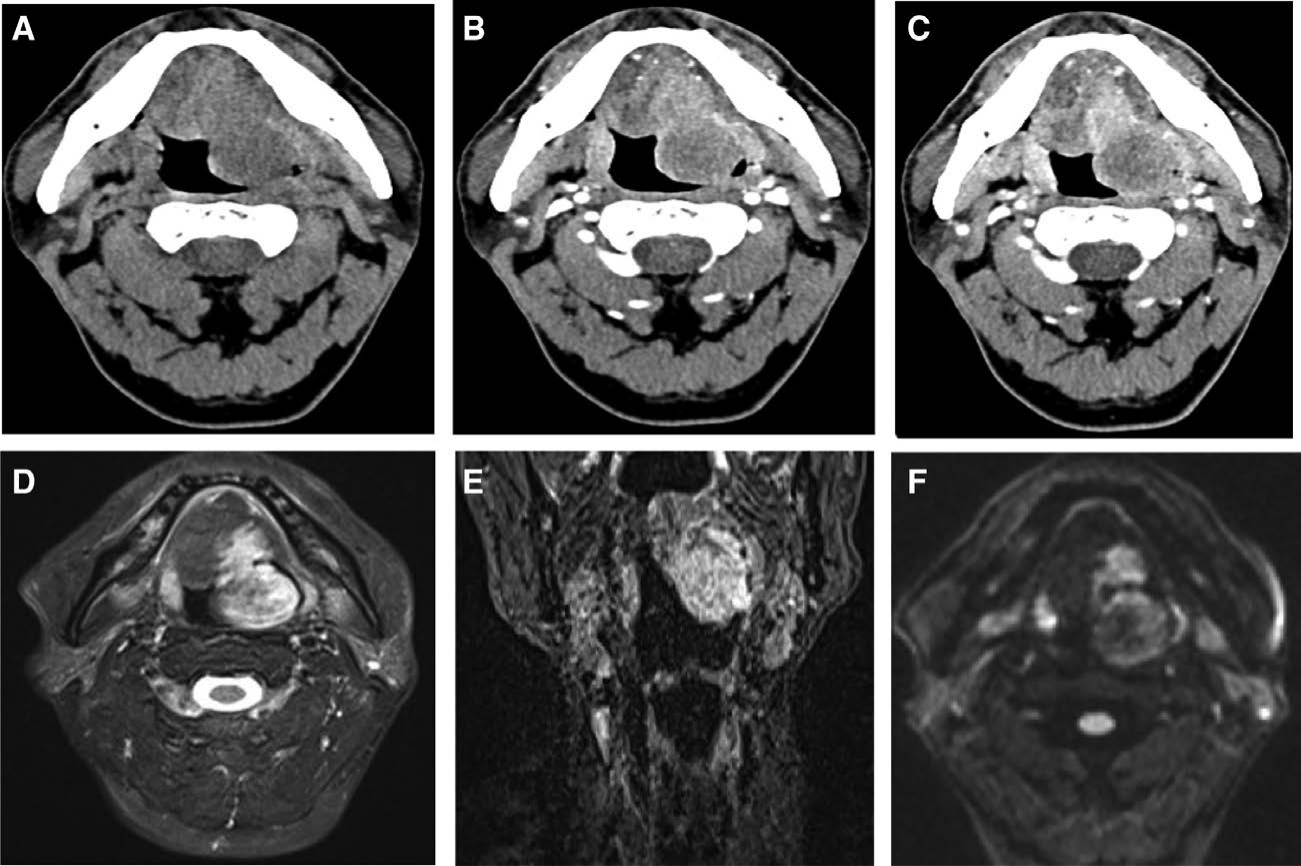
Pleomorphic adenoma of the left tongue CT scan, arterial phase and venous phase shows: irregular soft tissue density occupancy of the left tongue with heterogeneous density and progressive enhancement after enhancement, (A–C) axial compression lipid *T*2-weighted image sequence, (D) coronal compression lipid *T*2-weighted image sequence sequence, (E) Axial DWI sequence b = 1000mm/s^2^, and (F) shows: *T*2-weighted image sequence of the left tongue shows a heterogeneous obvious high signal occupancy, with linear low signal of each sequence visible at the edge and high signal on DWI. CT = computed tomography.
